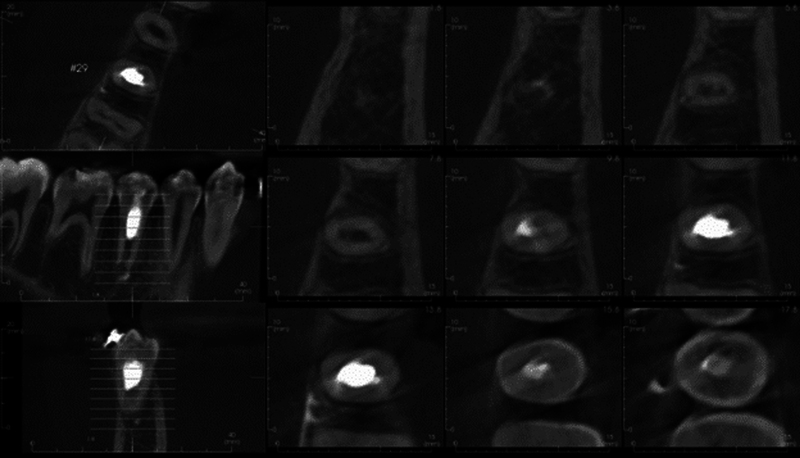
Multiple axial slices of cone beam computed tomography of tooth #29 displaying root endoclosure and no periapical pathology.
